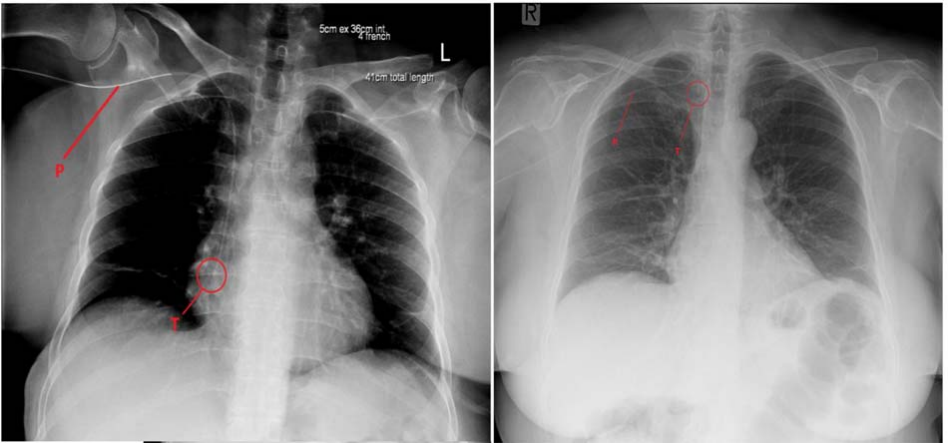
Chest X-ray after (left) PICC insertion and after withdrawal (right). P—PICC line tubing traced to the tip (T) in the right atrium (left) and superior vena cava (right).
